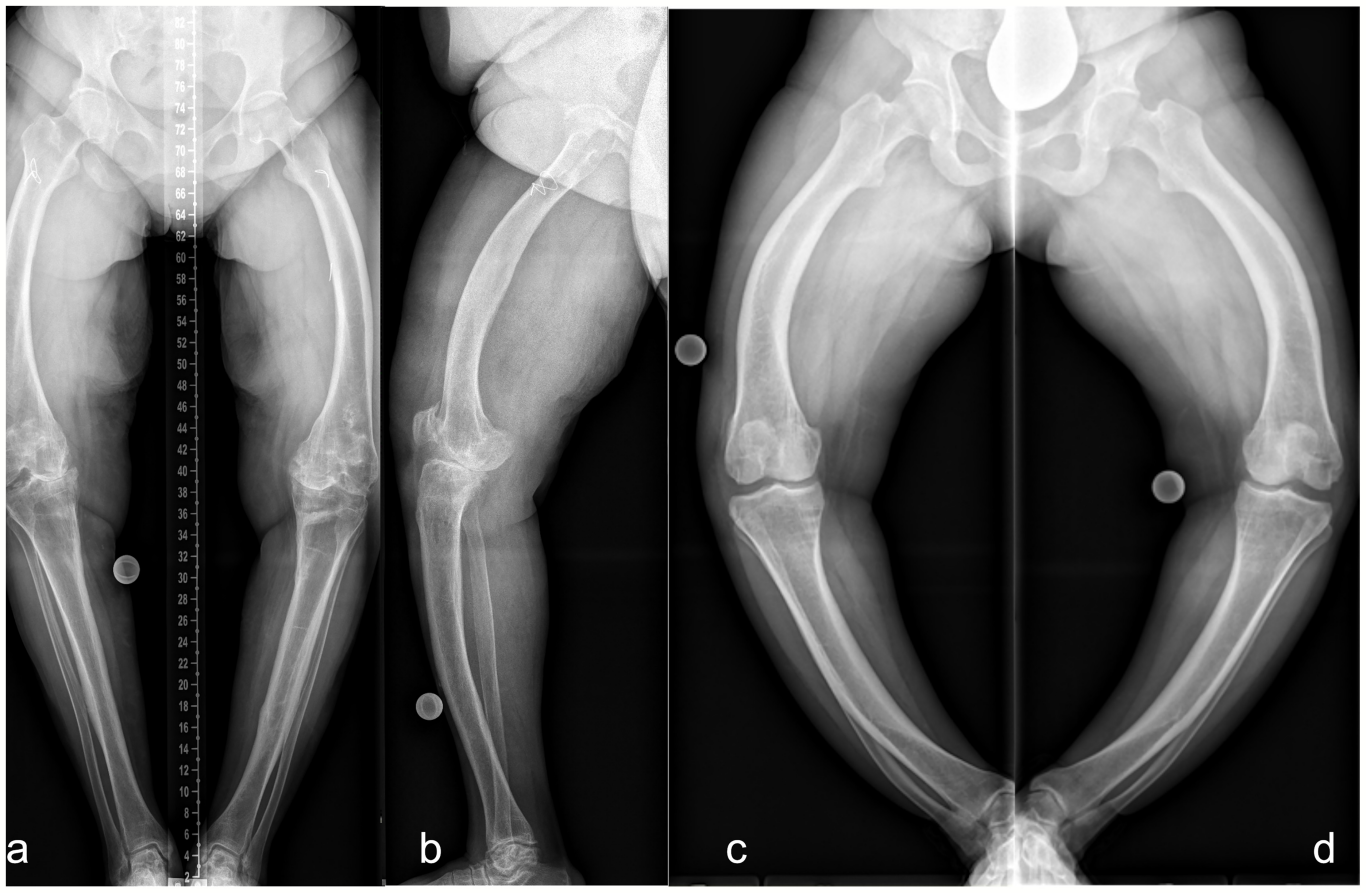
Lower limb deformity of patients prior to surgical frontal plane correction at our hospital. Left (A frontal, B sagittal): 50 y/o, female, five prior surgeries, GDI: 43.7, BMI: 28.7 kg/m^2^, conventional oral medication. Right: 33 y/o, female, no previous surgeries prior to presentation, GDI: 27.6, BMI: 37.6 kg/m^2^. Full-length anteroposterior radiograph (hip to ankle) of both lower limbs in standing position was not performed due to severe varus deformity. Thus, radiograph image of each leg was taken individually (C, D).
